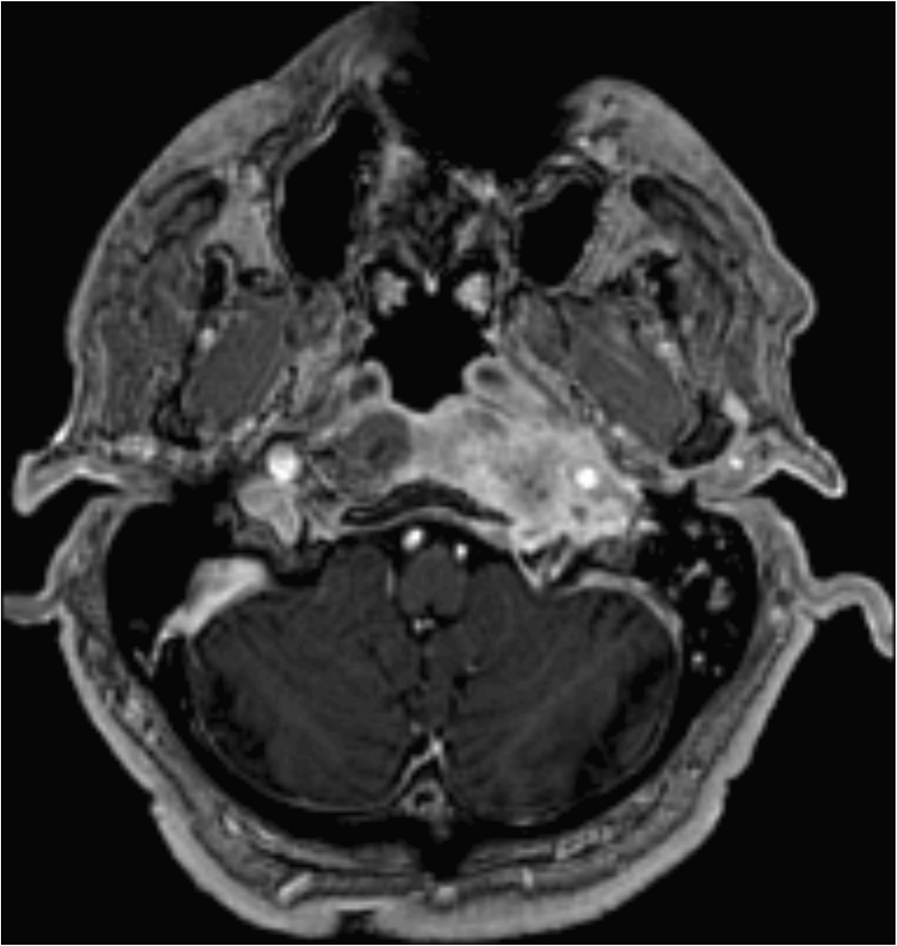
Day 5–75. Gadolinium-enhanced MRI, axial plane, T1-weighted, with fat suppression. Signal is homogeneously increased in the Eustachian tube and longus colli muscle. Cortical bone within the skull base is eroded, specifically the basal portion of the occipital bone ventrodorsally
